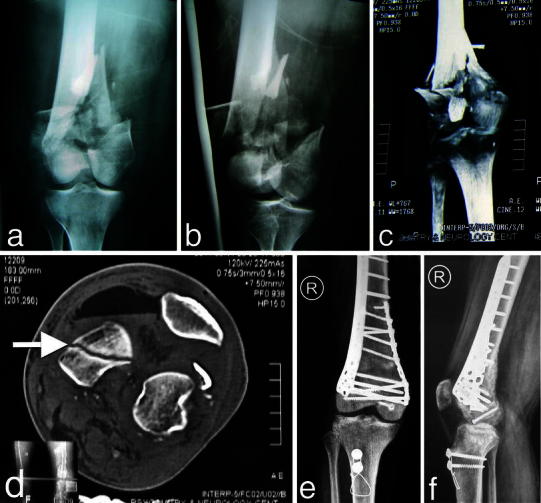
a, b Case number 11: AP and lateral plain X-ray views showing C3-type distal right femur fracture. c The related 3-D reconstruction anteroposterior CT scan. d The axial CT scan: distal cut showing medial Hoffa fracture (*white arrow*). The fracture was fixed by a lateral locked condylar plate, a medial contoured reconstruction plate, and a countersunk screw for the medial Hoffa fracture. e, f AP and lateral views 1 year after surgery, showing good union. The patient regained about 120° of knee flexion without any extensor lag
